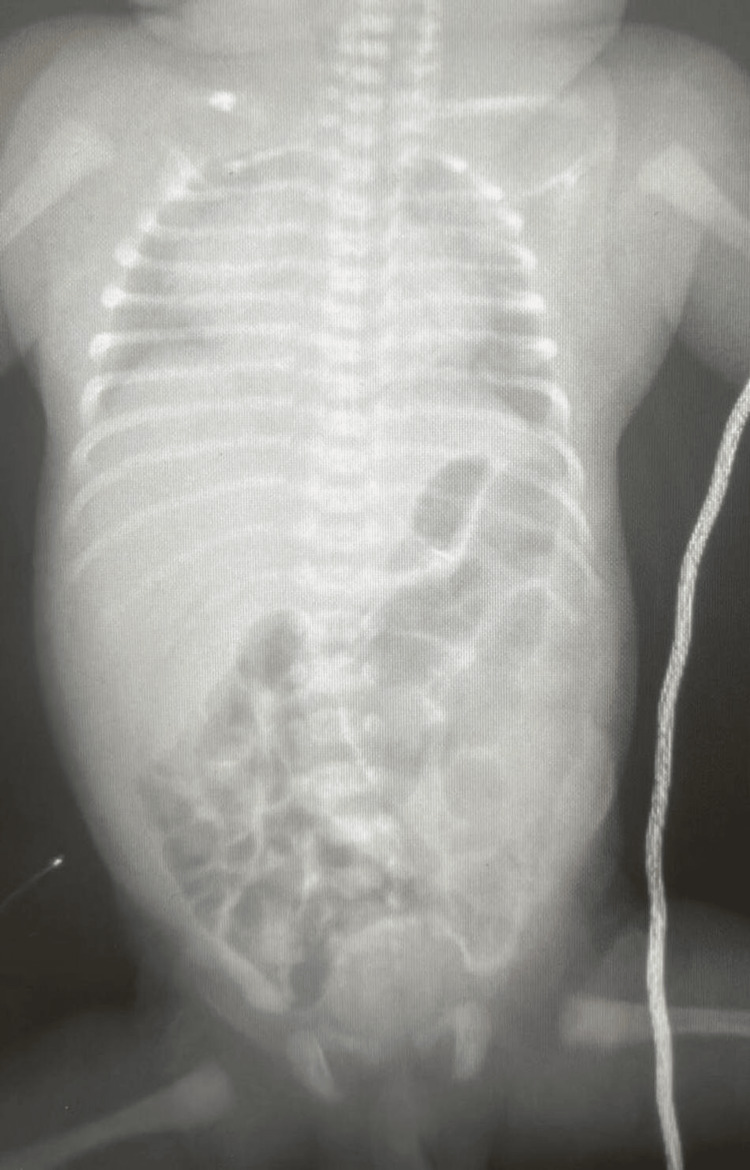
Admission chest X-ray revealed widespread pulmonary opacification and a modestly enlarged heart contour.
